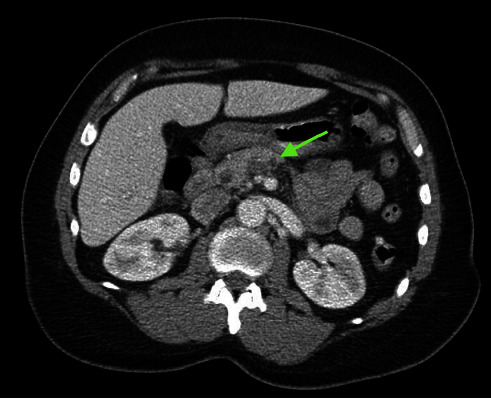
Axial section obtained from CT of the abdomen and pelvis showed findings consistent with superior mesenteric vein thrombophlebitis (green arrow).
